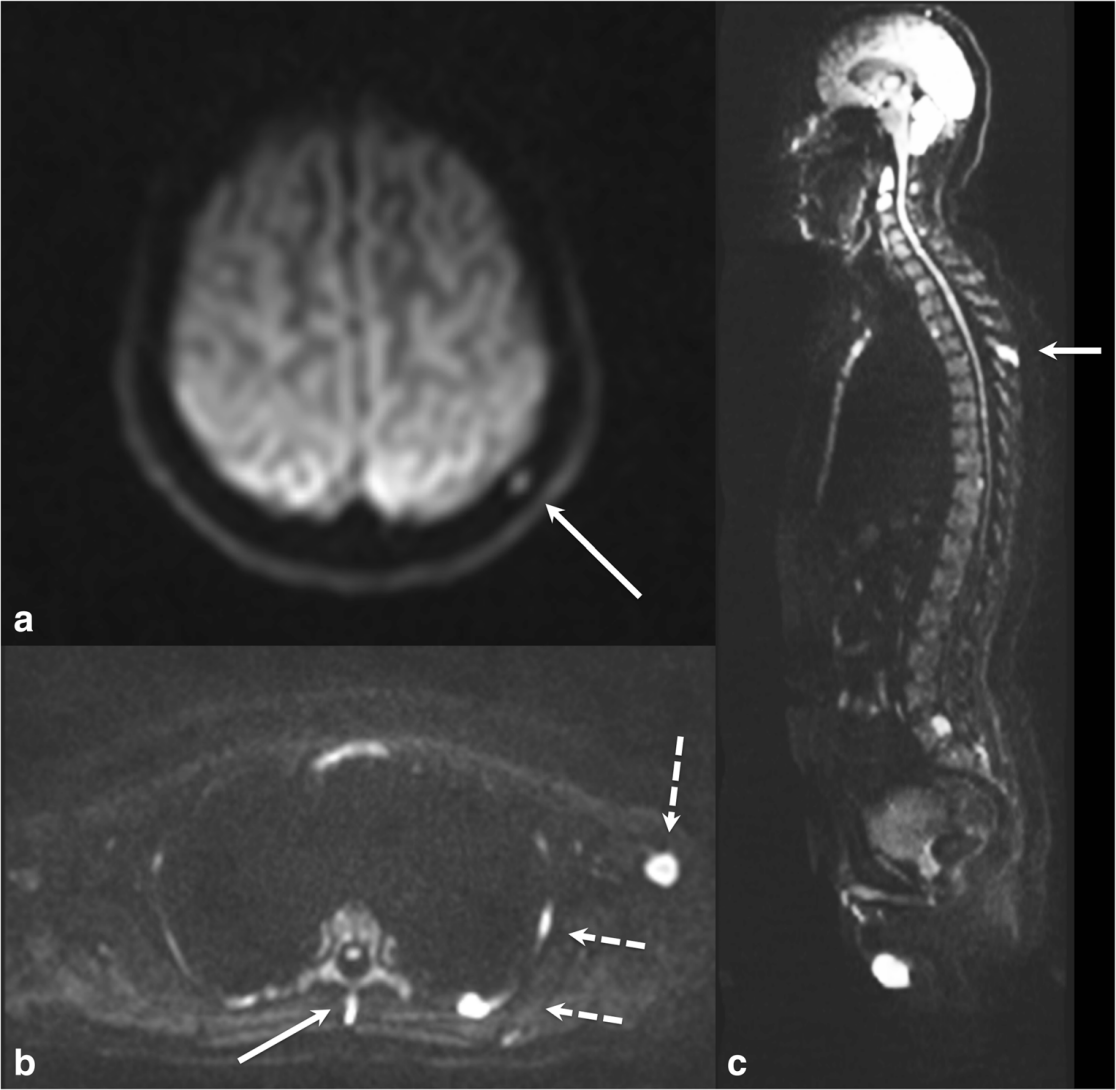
A 50-year-old gentleman with kappa light chain myeloma and 20% clonal cells on trephine bone marrow biopsy was found to have focal lesions in the cervical and lumbosacral spine, ribs and long bones on WB-MRI by all observers. The experienced observers detected additional small deposits in the left parietal skull vault (a) and in the spinous process of T4 vertebra (b, solid arrow) on an axial b900 DWI images; the latter being more evident on the b900 sagittal reformatted images (c, arrow). These subtle lesions were missed by the junior observers, resulting in a discrepancy in observer scores. The left humeral and left rib lesions (b, dotted arrows) were detected by all observers
